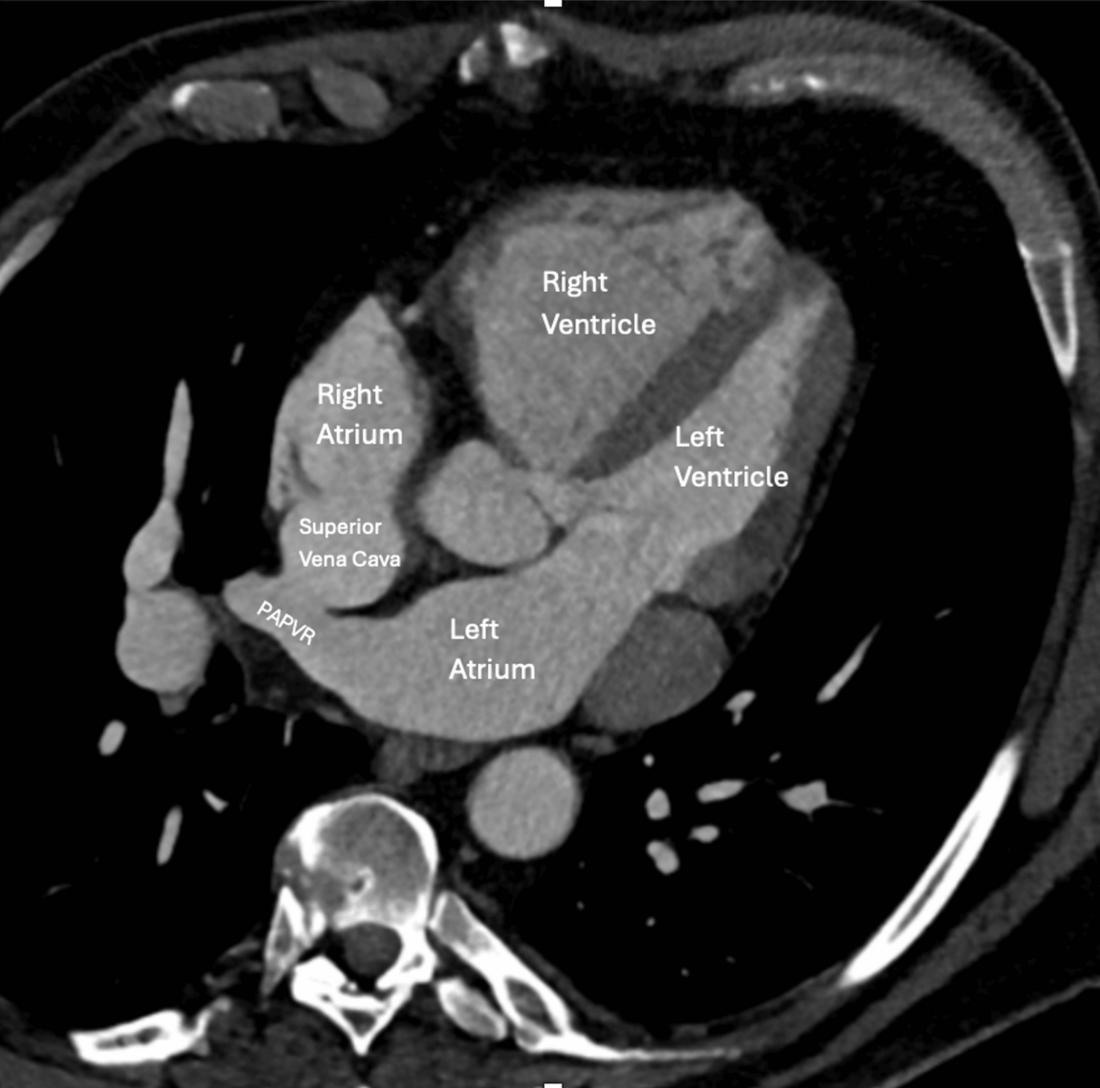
CT heart A contrast-enhanced CT of the heart showed four anomalous right pulmonary veins draining into the SVC, which was consistent with PAPVR. Additionally, a persistent left-sided SVC was noted, contributing to coronary sinus dilation. PAPVR: Partial anomalous pulmonary venous return; SVC: Superior vena cava
